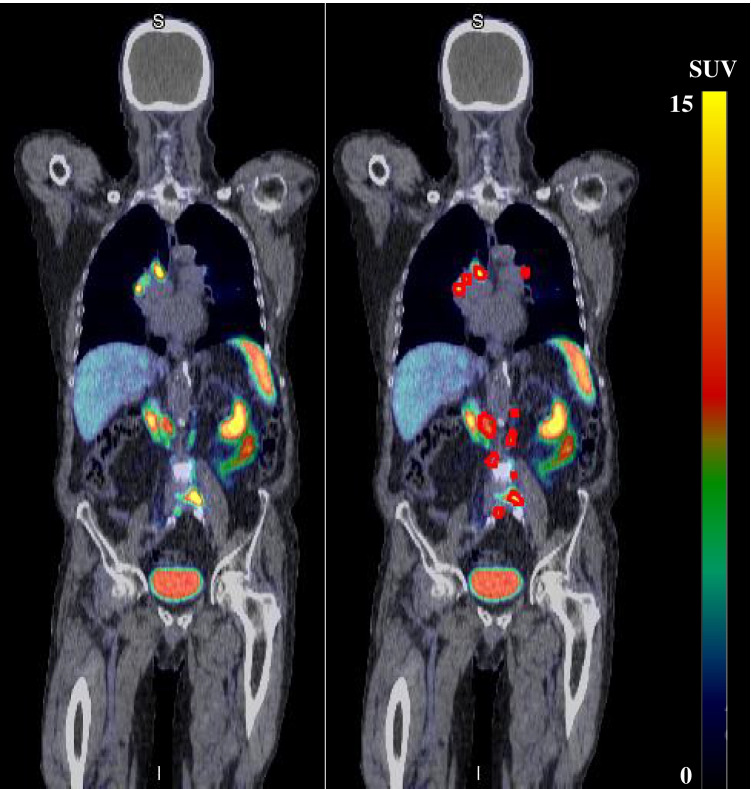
Exemplar whole-body [^68^Ga]Ga-PSMA PET/CT fusion scan acquired from the middle of the thigh to the skull vertex for a single patient. Screenshots were extracted from the MIM Encore software used for delineation and visualisation. On the left, a coronal slice from the PET/CT fusion is shown without any contouring, and on the right the same coronal slice is displayed with metastatic PCa lesions delineated clearly in red
